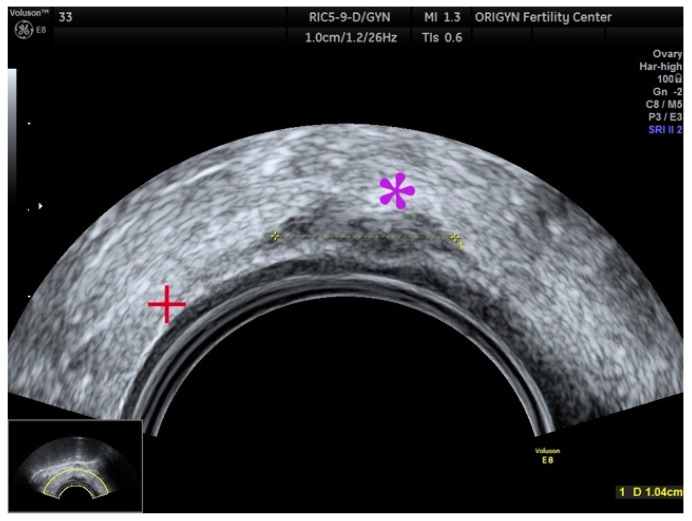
Ultrasound image depicting an endometriotic nodule of the RVS. (+) the vaginal wall as a hypoechogenic line and an endometriotic nodule (*) with discreet vaginal wall infiltration.
